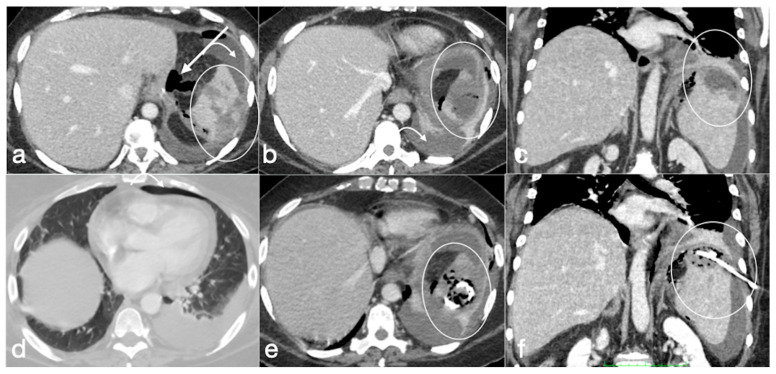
Patient 2. Suture leak with spleen abscess after sleeve gastrectomy. A 48-year-old patient underwent sleeve gastrectomy and complained in the following days of abdominal pain, fever, and dyspnea. At the first CT examination is seen the leak (a, axial view, straight arrow), free peritoneal fluid (a, curved arrow) and inhomogeneous enhancement of the spleen (a, circle). In more cranial scans, a splenic abscess is seen (b, circle) associated with the reactive pleural effusion (b, curved arrow), and the coronal view (c) further clarifies the finding (c, circle, splenic abscess). It was decided that the patient should be managed with percutaneous drainage of the splenic abscess. The patient came back after the drainage positioning, with chest pain and dyspnea (d–f) and at CT the left anterior pneumothorax (d, axial view, curved arrow) was detected due to the drainage positioning, which is actually in the splenic abscess (e, axial view, circle). However, in its course it crosses the left diaphragm (f, coronal view, circle).
